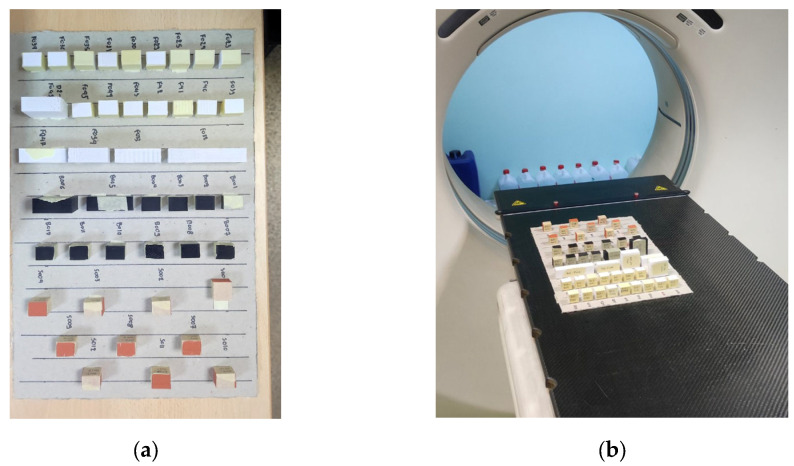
(a) 3D-printed calibration cuboids for testing the proposed method, placed on a CT scanner bed (b).
